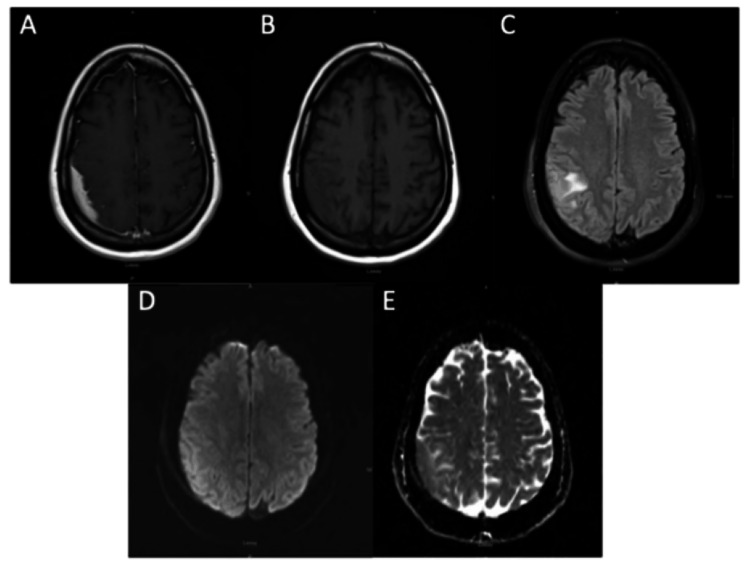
Multiple MRI sequences revealing extra-axial collection within right parietal convexity (A) Contrast-enhanced axial MRI showing homogeneously enhancing extra-axial collection within the right parietal convexity. Mixed isointense to hyperintense signals were appreciated on T1 (B) with slight hyperintense signals on T2 (C) with surrounding vasogenic edema, DWI hyperintensity (D), and ADC isointensity (E).
